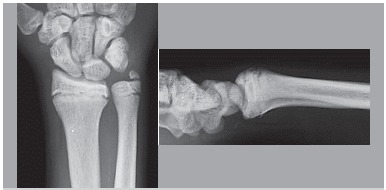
Front and profile x-ray of the right wrist of a young athlete with complain of wrist pain. Observe the enlargement of the distal physis of the radius and ulna and blurring of the radiolucent physis area.^11^
